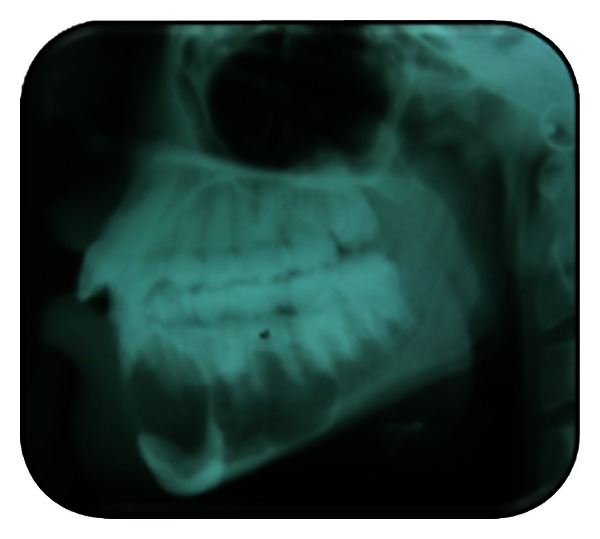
Lateral cephalogram radiograph.
